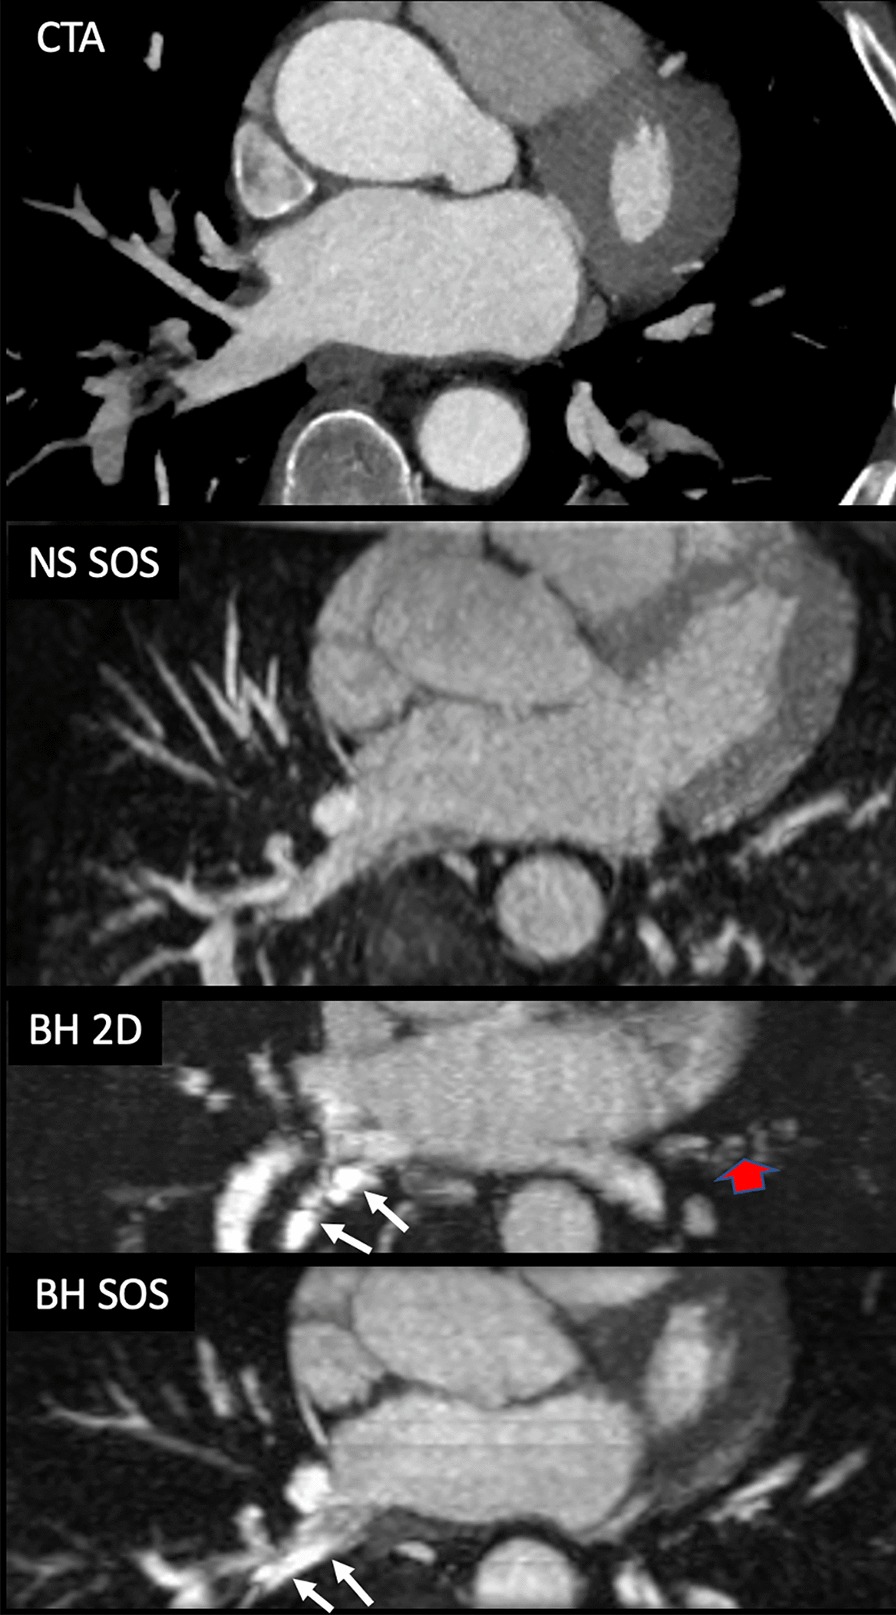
Example of a subject undergoing evaluation prior to radiofrequency catheter ablation with CTA and non-contrast CMRA. Axial maximum intensity projections are shown, reconstructed from the coronal acquisitions. Note the off-resonance artifact seen at the level of the right inferior pulmonary vein ostium on both BH-2D and BH-SOS, which degrades the image quality and sharpness of the vessel contour. There is also some residual misregistration artifact with BH-2D (wide red arrow). However, the ostial diameter can still be adequately measured. NS-SOS shows sharp margins of the right inferior pulmonary vein ostium with negligible off-resonance artifact
